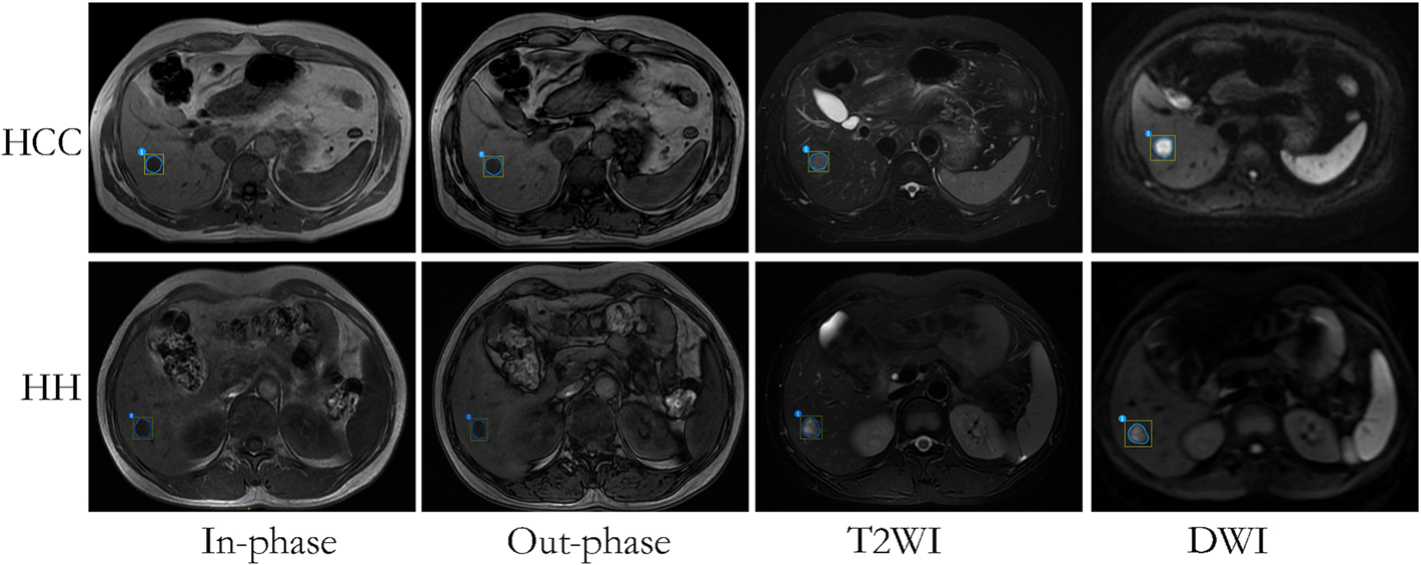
Imaging segmentation on HCC and HH. The ROIs which enclose the boundary of target lesions on in-phase, out-phase, T2WI and DWI are depicted
